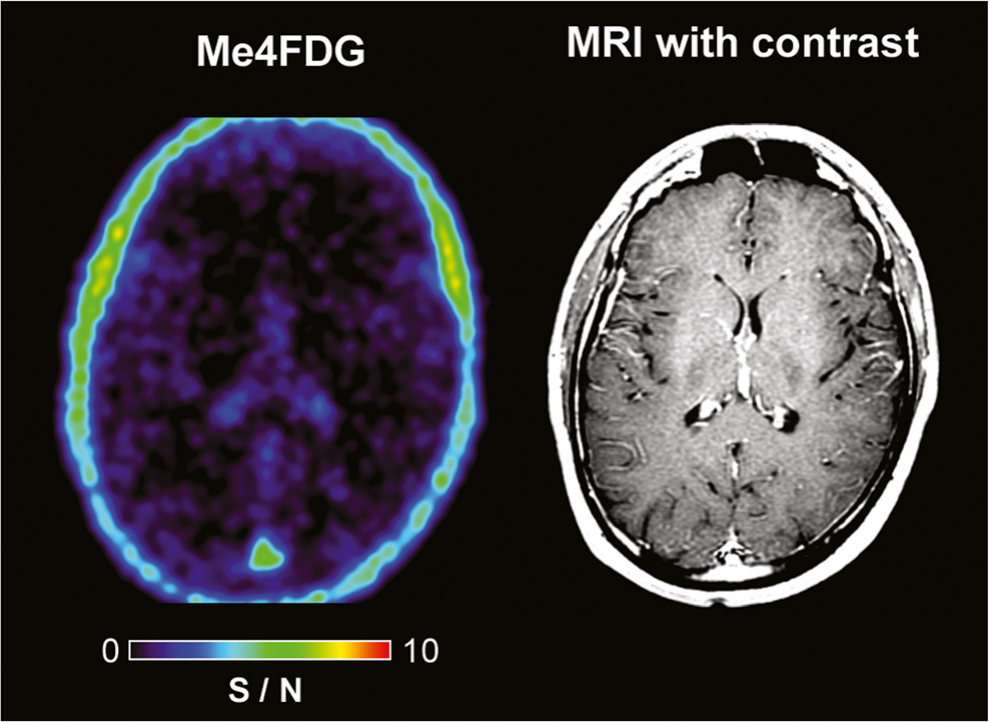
Me-4FDG and MRI scans on a control subject. Scans were conducted on a 24-year-old male patient with a history of epilepsy. The MRI (T1-weighted MP-RAGE with and without gadolinium contrast) was carried out as part of his clinical care. The brain Me4FDG PET scan 30 min after intravenous injection of 370 MBq Me4DG was conducted on a Siemens Biograph PET/CT scanner. The SUVR/BG scale, 0–10, is shown for the NIH color scale. Revised from Kepe et al. [10]
